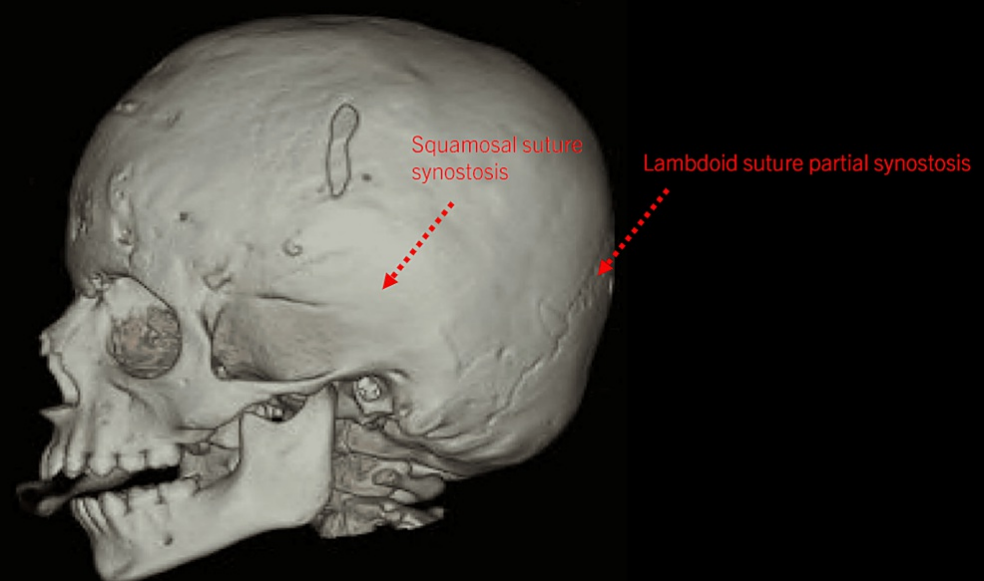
CT scan and three-dimensional reconstruction of the skull post anterior cranial vault remodeling with good expansion of anterior vault but with subsequent lambdoid synostosis and flattening of occiput.
